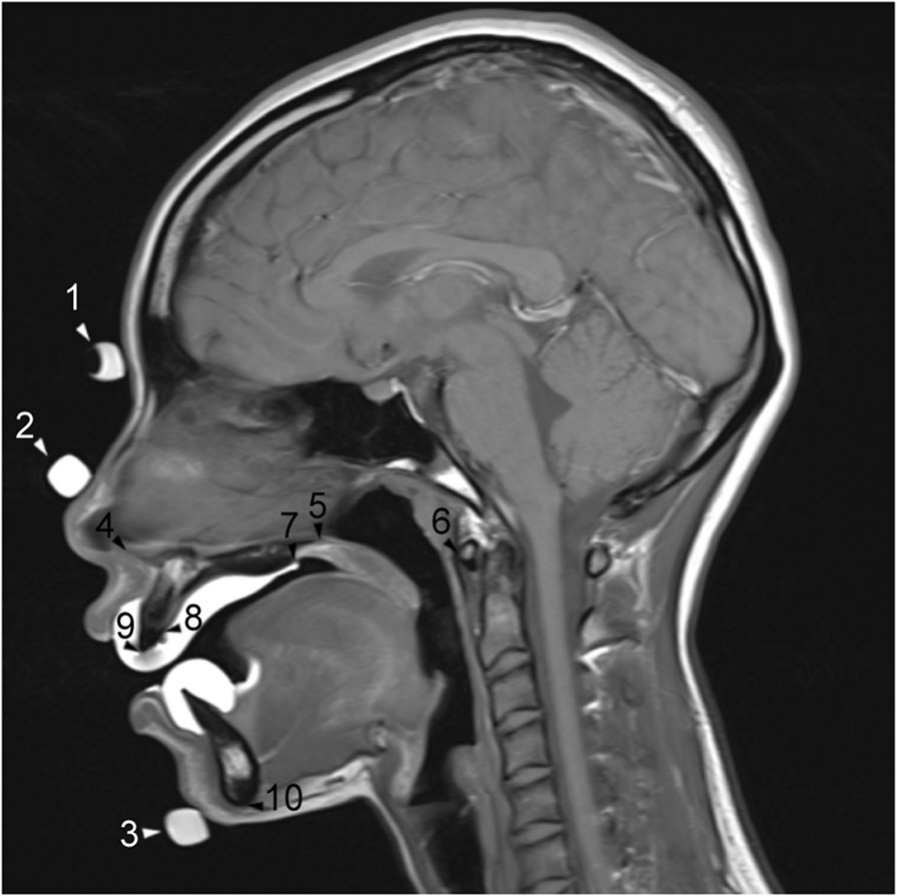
A representative static magnetic resonance image. Landmarks on the (1) forehead, (2) nasal tip, (3) chin, (4) ANS (anterior nasal spine), (5) PNS (posterior nasal spine), (6) C1 (the most anterior point of the atlas), (7) PM (point at which the soft and hard palates intersect), (8) AM (boundary point between the maxillary central incisor and the palatal mucosa), (9) I (point at the edge of the maxillary incisor), and (10) Me (the most posterior point on the mandibular symphysis). For abbreviations, see Table 1
